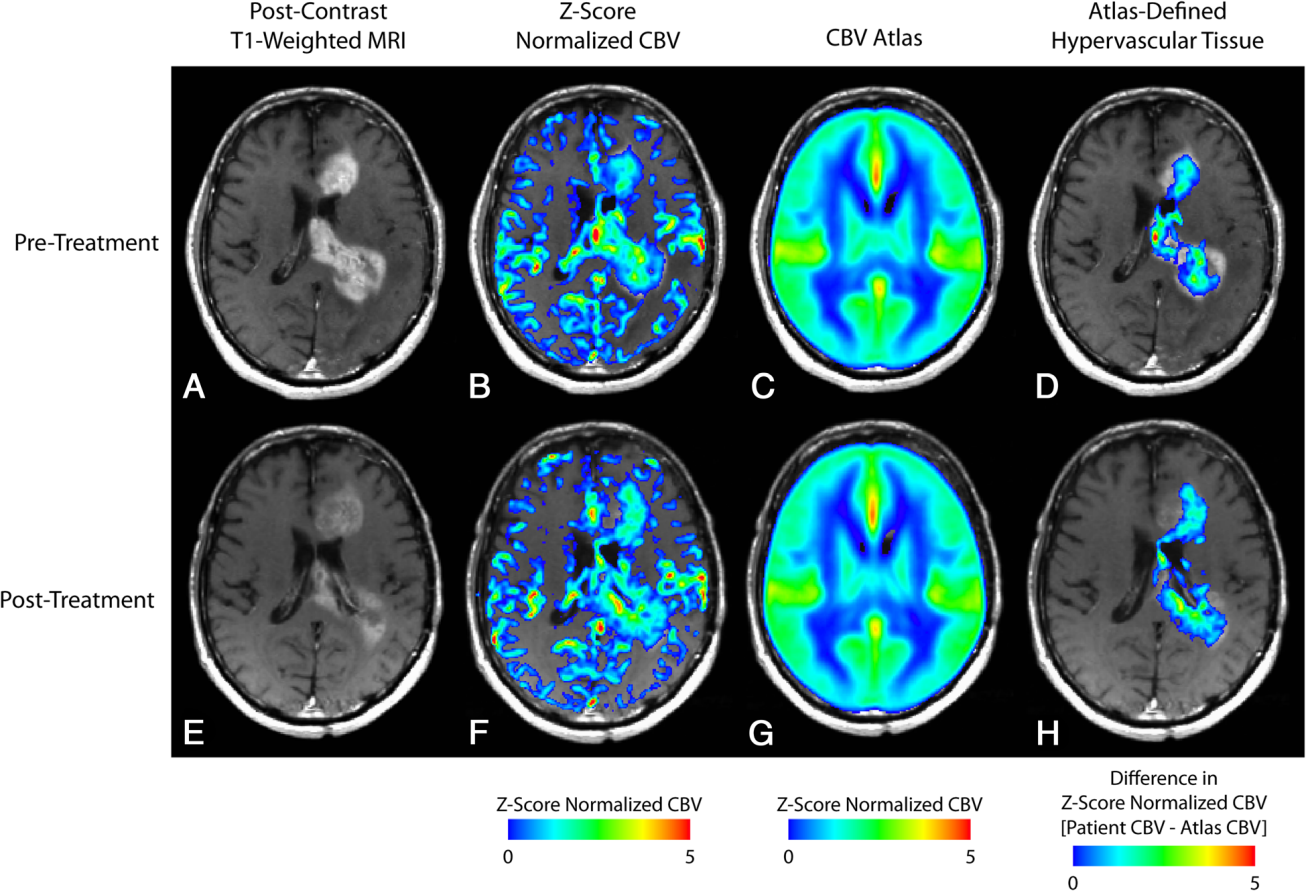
Non-responder to bevacizumab (PFS =36 days; OS =62 days). A) Pre-treatment, post-contrast T1-weighted image. B) Pre-treatment, Z-score normalized CBV. C) CBV atlas. D) Pre-treatment, atlas-defined hypervascular tumor tissue showing a large extent of abnormal vasculature prior to therapy. E) Post-treatment, post-contrast T1-weighted image. F) Z-score normalized CBV. G) CBV atlas. H) Post-treatment, atlas-defined hypervascular tumor tissue showing a large residual volume of abnormal vasculature after administration of bevacizumab.
